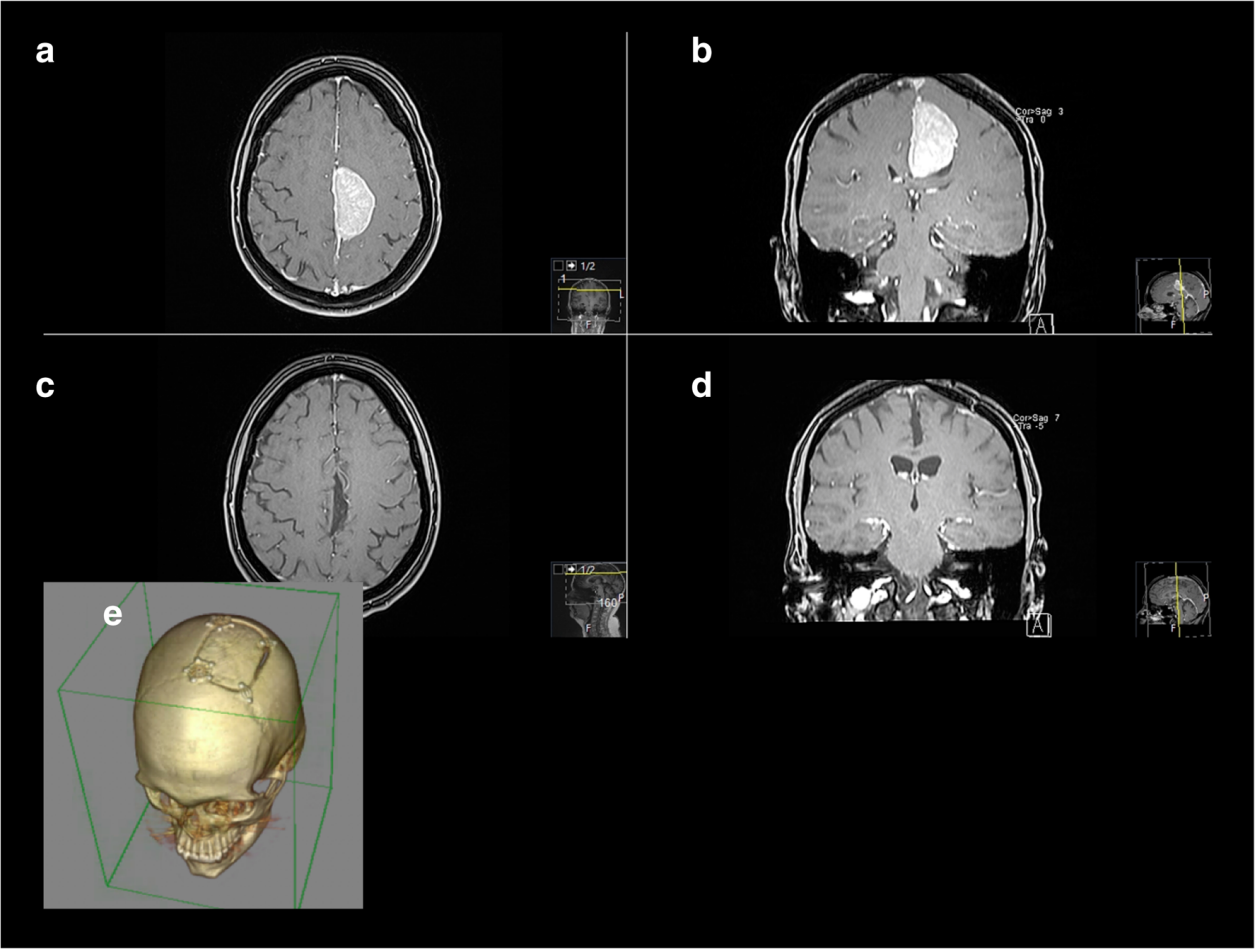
Exemplary case 1: A 35-year-old female patient presented with depression and seizures. a, b Preoperative T1-weighted gadolinium–enhanced MR imaging showing a left-sided falcine meningioma. c, d Postoperative MR control showing complete removal, Simpson I. Pathologic findings revealed atypic meningioma WHO grade II. e Postoperative 3D-CT reconstruction showing the bone flap for a FIA
